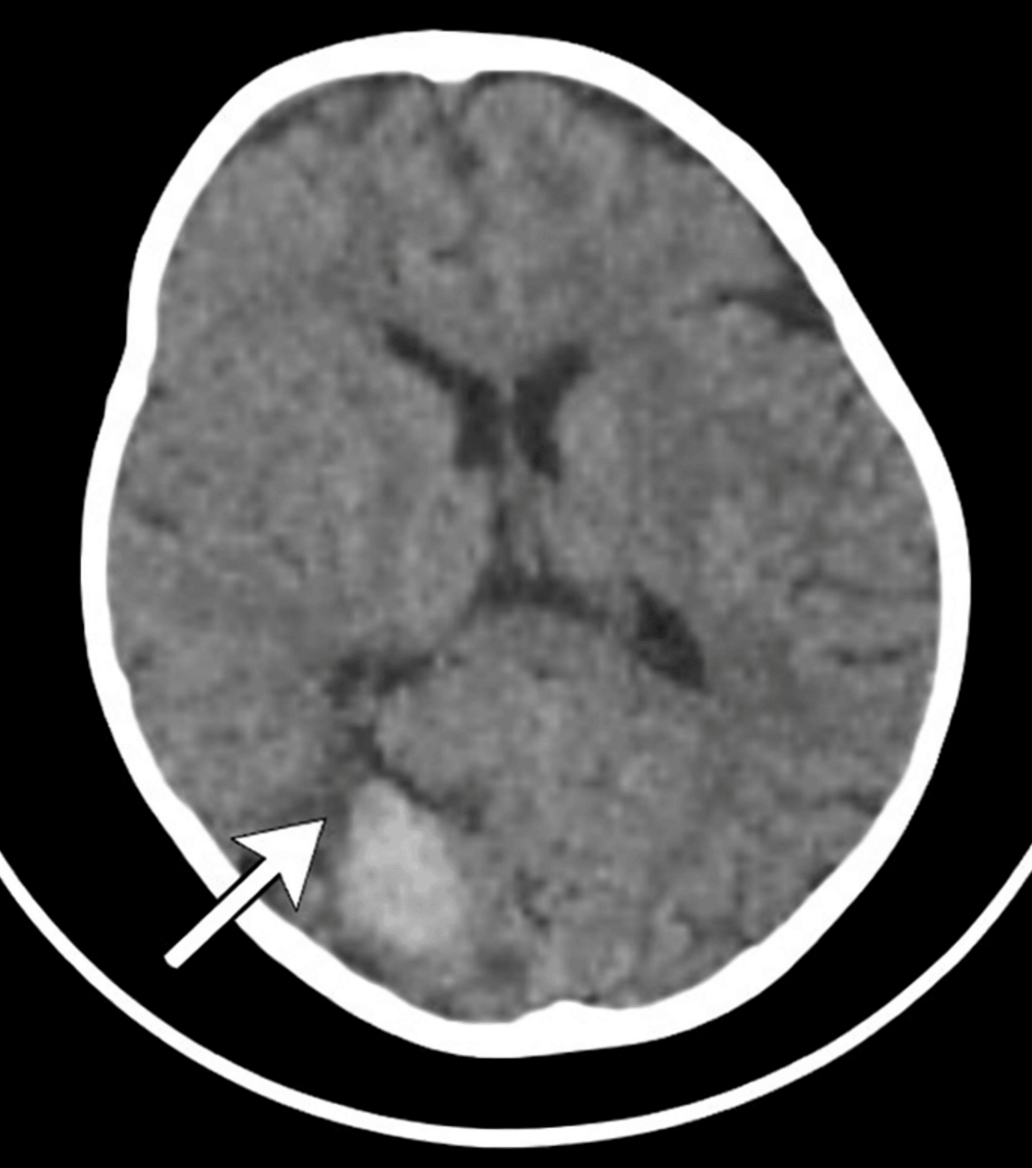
A brain CT scan revealed a right occipital intraparenchymal hematoma with a mass effect
